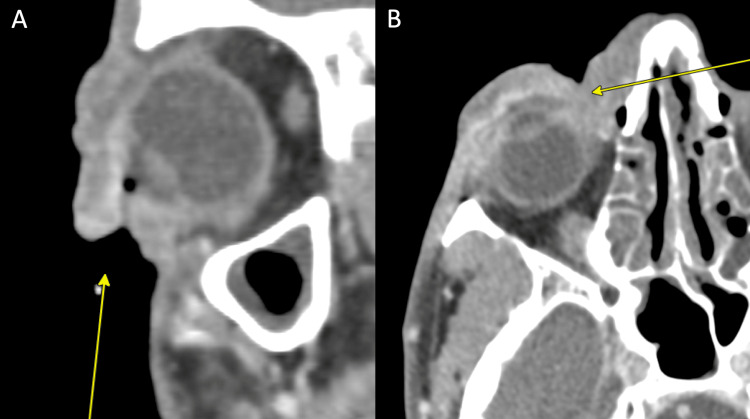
Coronal (A) and axial (B) CT images of the maxillofacial regions demonstrating soft tissue swelling over the left frontal bone and left temporal region. CT: computed tomography
